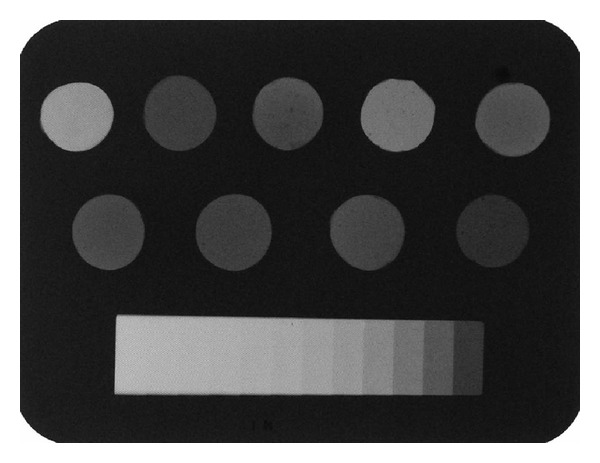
Radiographic film obtained with one disc of each material and the graduated aluminum stepwedge.
